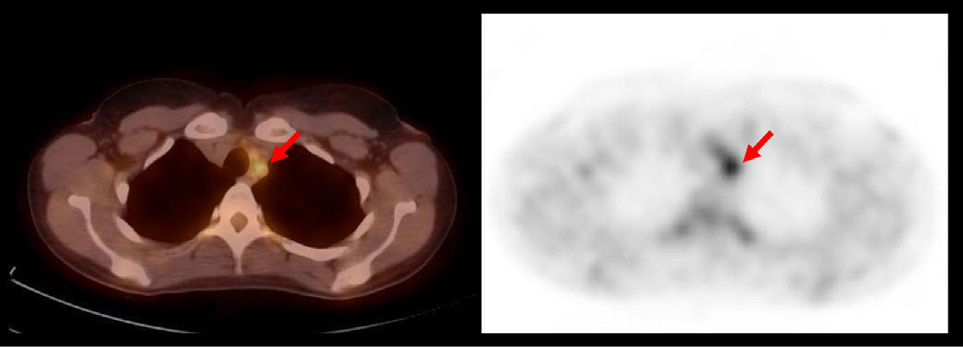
This 38-year-old woman presented with severe headaches. MRI revealed a swollen right hemisphere and impaired cerebral perfusion. Treatment with steroids was initiated. PET/CT revealed an enhancement of the supra-aortic arteries suggestive of a large-vessel vasculitis (red arrows). Takayasu arteritis was diagnosed.
